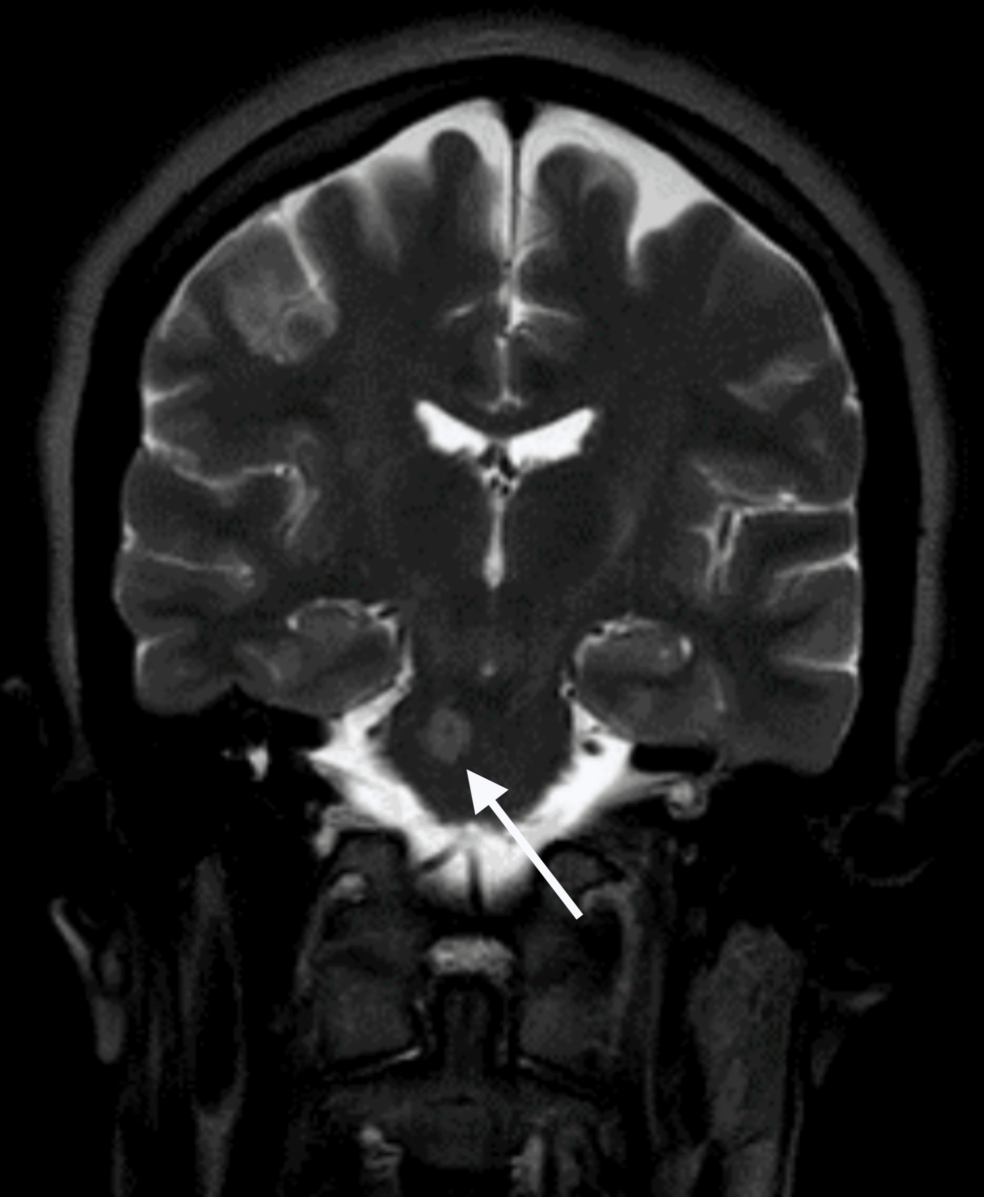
Brain MRI performed postictal (coronal section, T2 imaging) The same right lateral pontine lesion, as seen in Figure 3, with T2 hyperintensity (white arrow) MRI: magnetic resonance imaging
